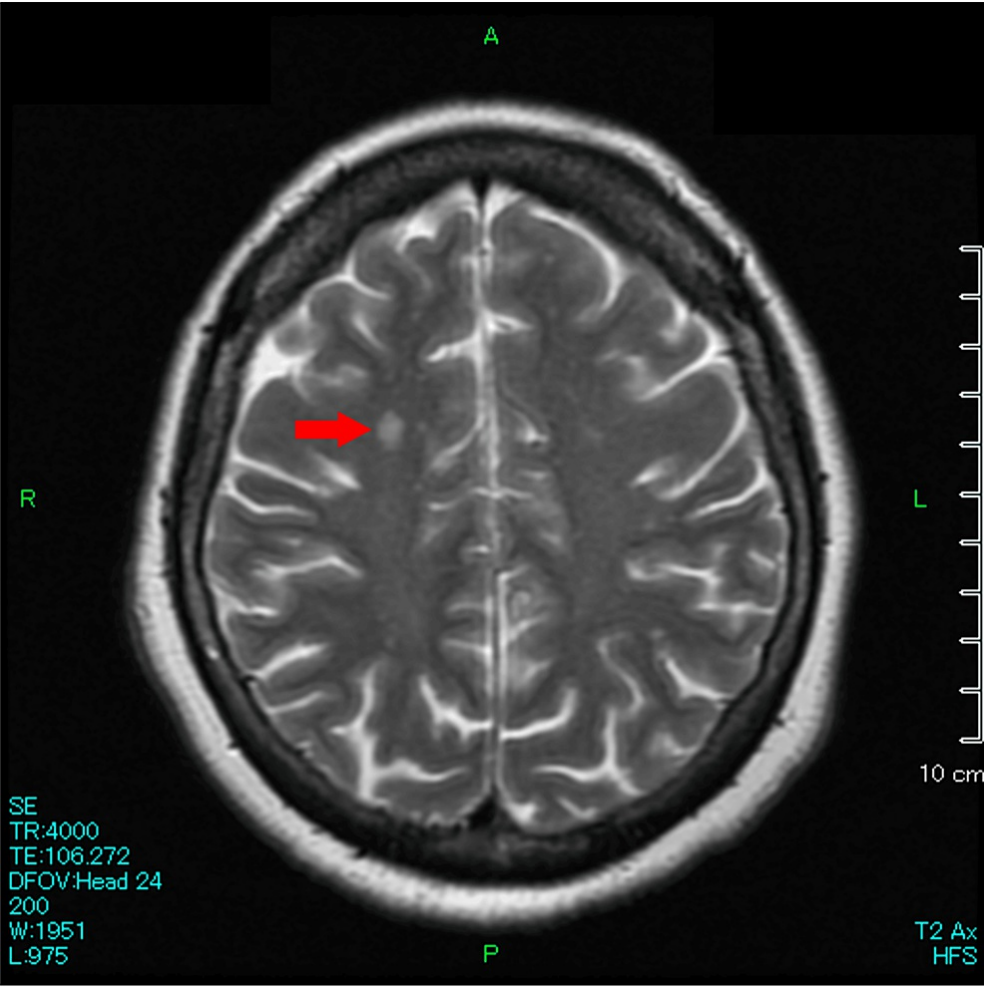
Magnetic resonance image (MRI) of the brain showing T2 hyperintensities in the cerebral white matter.
